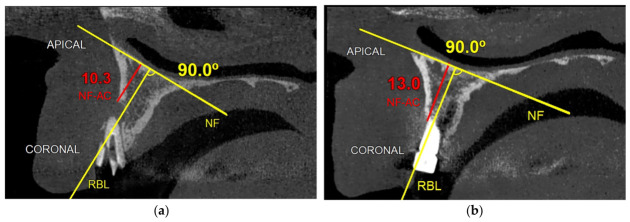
Standardized sagittal CBCT sections for vertical morphometric assessment at baseline (T0; a) and 12-month follow-up (T1; b). The nasal floor (NF) was used as the anatomical reference; the reference baseline (RBL) was drawn perpendicular to the NF tangent, and the nasal floor–alveolar crest distance (NF–AC) was measured along the RBL.
